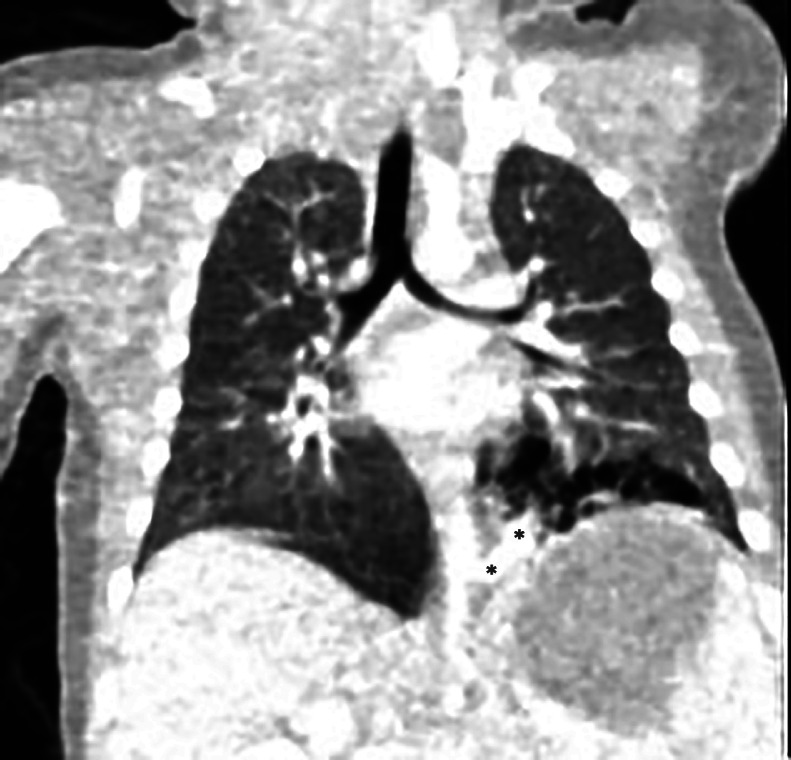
Coronal CT angiogram at the level of the aorta depicting the feeding vessel of the BPS and (marked with black asterisks) and air-filled CPAM component the hybrid lesion of the left lower lobe.
